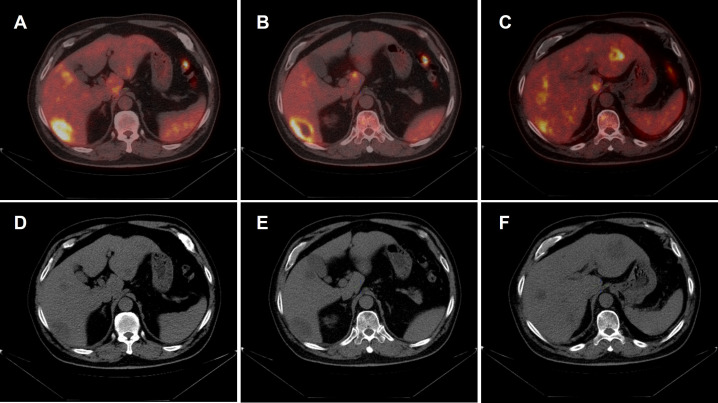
^18F-FDG PET/CT findings. (A–C) Fused PET/CT images demonstrate multiple intrahepatic hypermetabolic nodules with ill-defined margins. The largest lesion measures approximately 4.7 × 2.7 cm, with a maximum standardized uptake value (SUVmax) of 9.9. Central photopenic areas are observed in larger lesions, suggestive of internal necrosis. (D–F) Corresponding non-contrast CT images show multiple low-density nodules.
